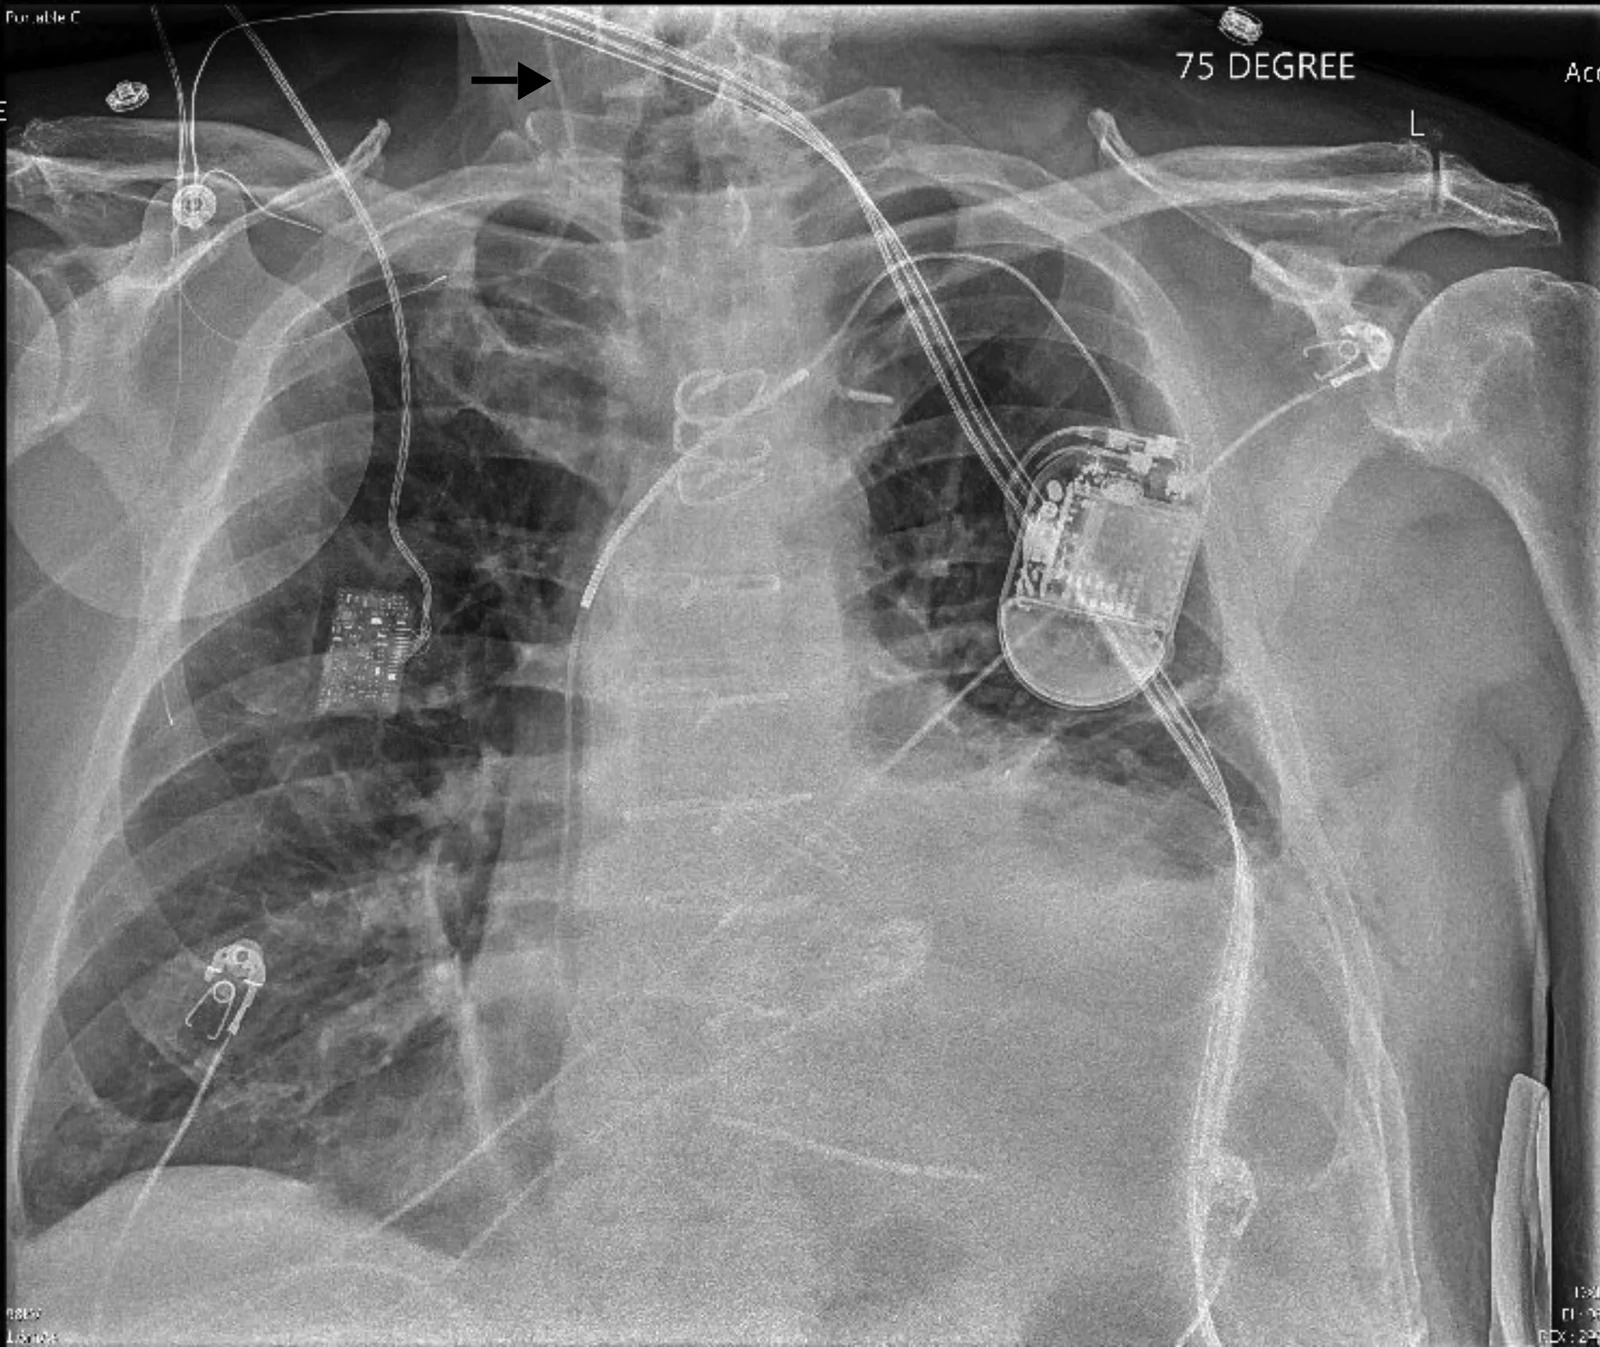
Anterior–posterior chest radiograph taken on postoperative day 8 (6 h after prior radiograph) showing peripherally inserted central catheter malposition with an upward migration into the right internal jugular vein (black arrow).
